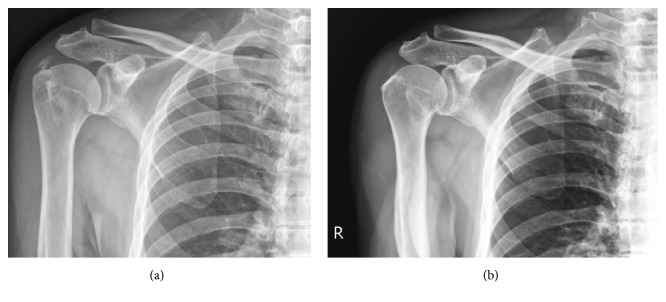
Application of ESWT on type II calcific shoulder tendinosis. (a) Plain film before ESWT (b) 6 months after ESWT. Complete resorption of calcium deposits with improvement of CMS from 60 to 88 and VAS from 5 to 1.
